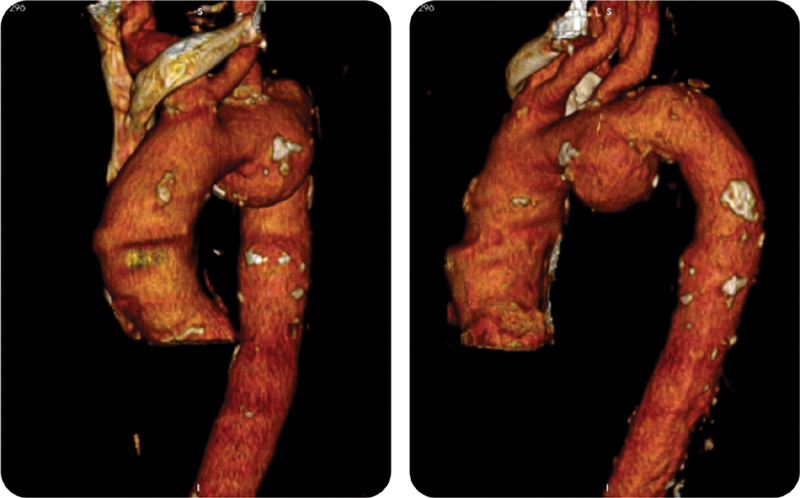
Preoperative computed tomography assessment scan.
